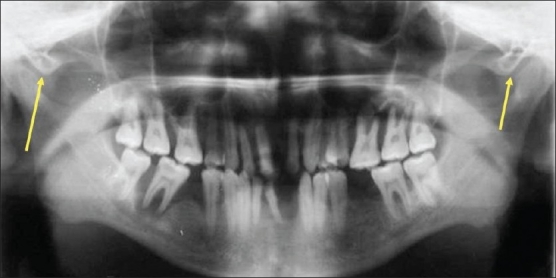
Panoramic radiograph shows bilateral multilocular ZACDs (arrows)
